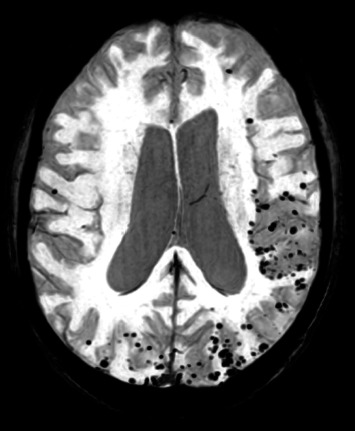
Gradient echo (GRE) MRI sequences demonstrating diffuse atrophy and multiple microbleeds.
